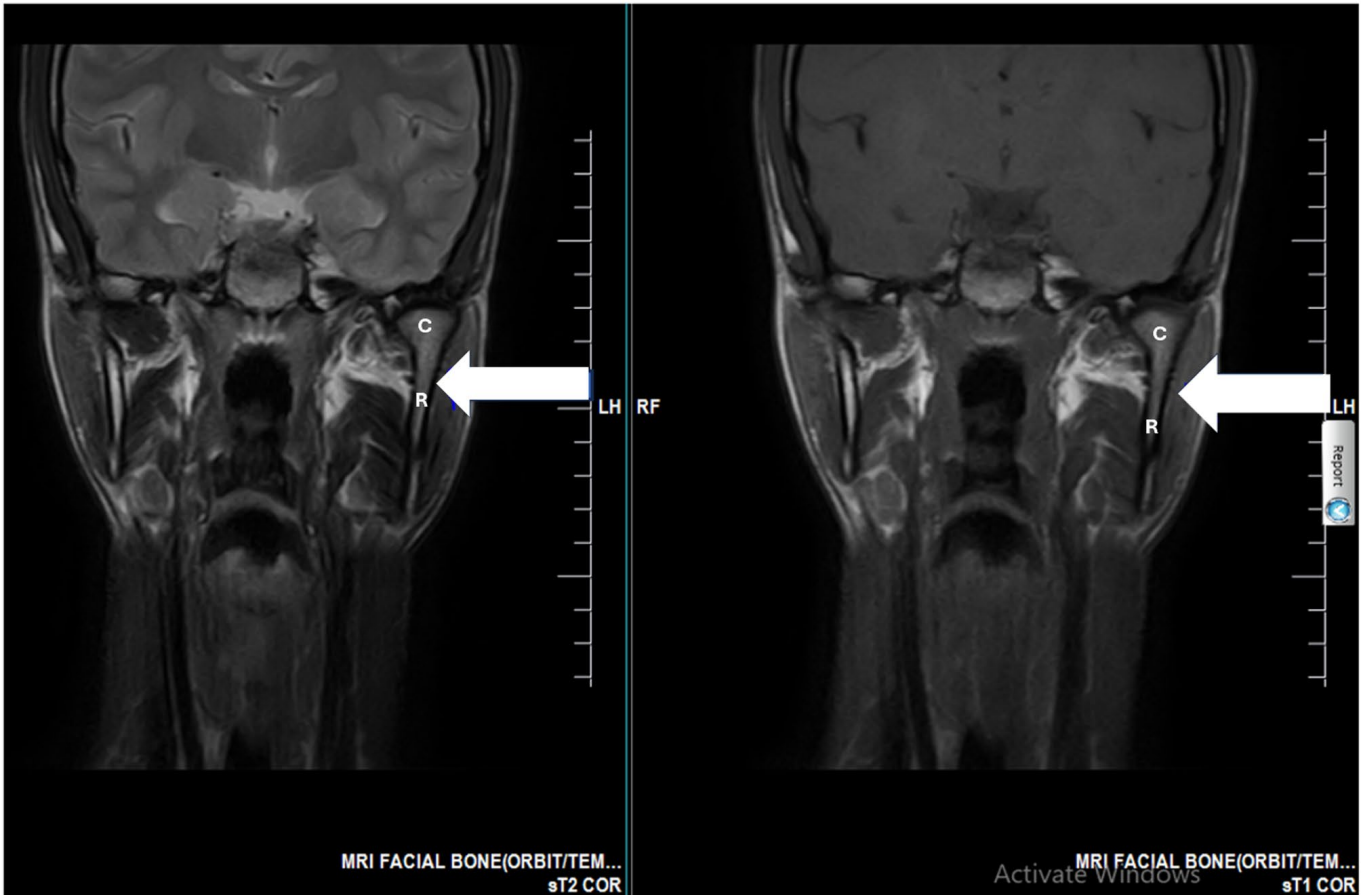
Coronal MRI showing left unilateral mandibular condylar hyperplasia. T2-weighted (Left) and T1-weighted (Right) coronal images showing asymmetrical thickening and elongation of the ramus and condyle of the mandible on the left side (white arrowhead, C = Condyle, R = Ramus) as the primary underlying pathology, when compared to the normal right side
